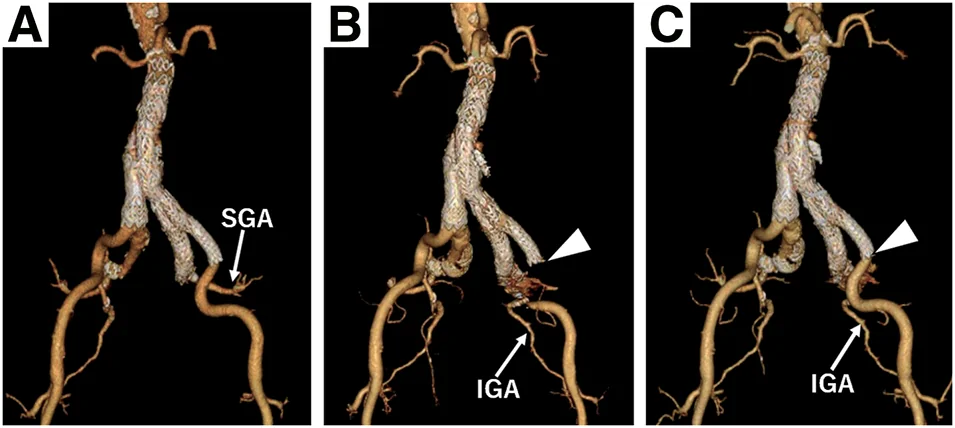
CT findings. Contrast-enhanced CT performed on postoperative day 7 demonstrates patency of both the internal and external iliac limbs (A). CT performed on postoperative day 16 reveals complete occlusion of both the internal and external iliac limbs of the iliac branch endoprosthesis (arrowhead). Distal perfusion of the external iliac artery is preserved via collateral circulation, and the inferior gluteal artery is opacified through collateral pathways (B). CT performed after reintervention demonstrates restored patency of the external iliac limb (arrowhead), whereas the internal iliac limb remains occluded. The inferior gluteal artery continues to be opacified via collateral circulation (C). IGA, inferior gluteal artery; SGA, superior gluteal artery; CT, computed tomography
